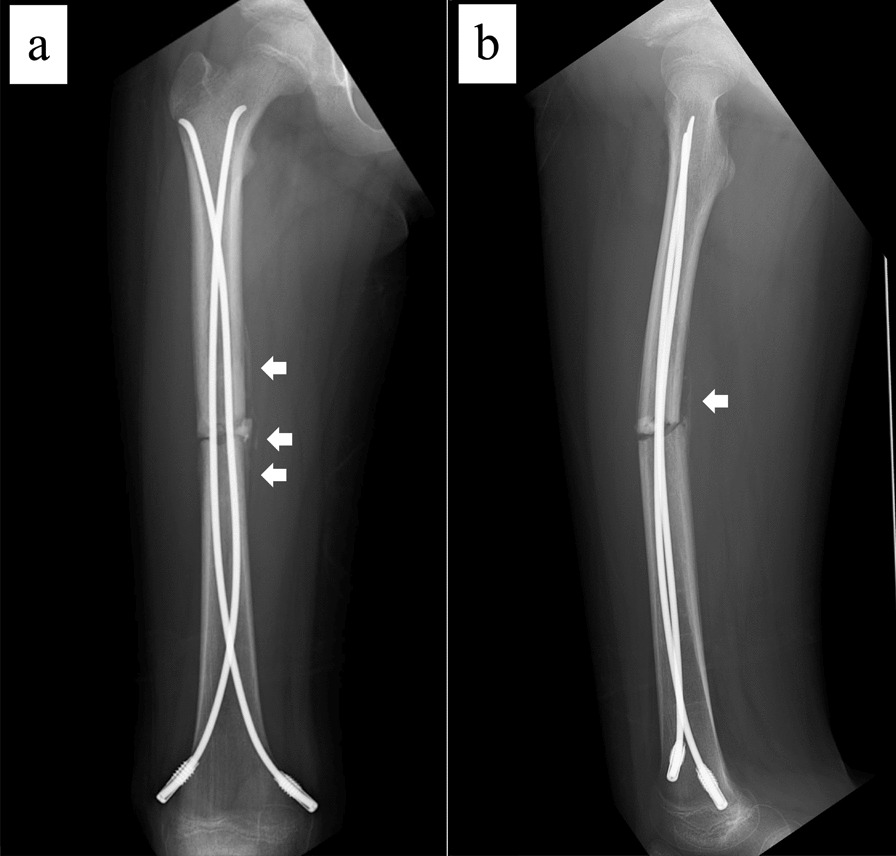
X-ray images at 5 weeks postoperatively. a Anteroposterior image and b lateral image. Callus formation is observed on the medial and posterior sides of the fracture site (white arrows)
